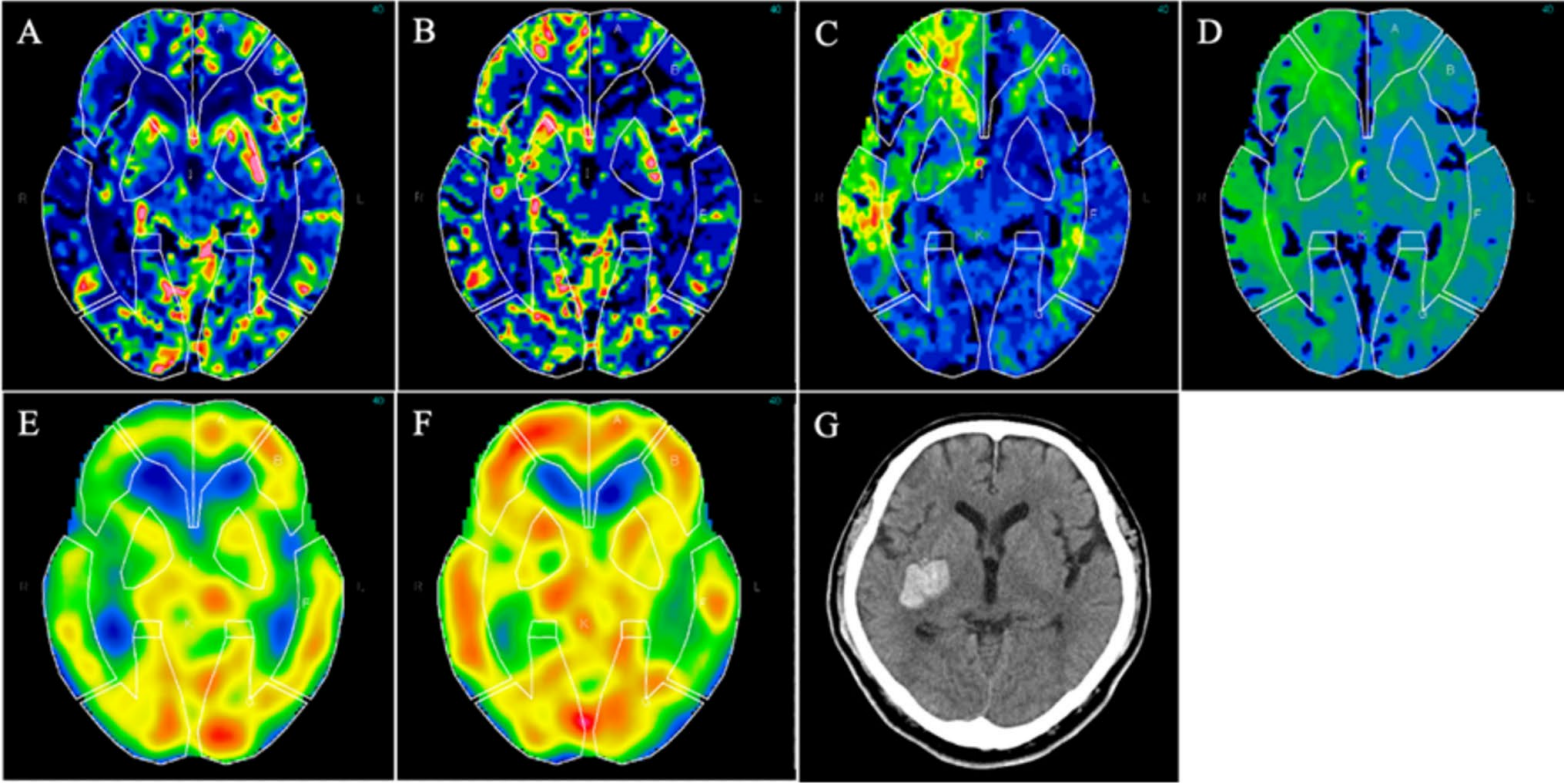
Imaging results of a 65-year-old man with a right carotid artery stenosis followed by intracerebral hemorrhage after CAS. Reconstructed maps of preoperative CTP showing a relatively decreased regional CBF (CBF ratio: 0.83) (A), increased regional CBV (CBV ratio: 1.24) (B), increased regional MTT (MTT ratio: 1.31) (C), and increased regional TTP (TTP ratio: 1.10) (D) in the right hemisphere. Preoperative ^123^I-IMP SPECT at rest showing decreased regional CBF (AI: 0.88) in the right hemisphere (E). Postoperative ^123^I-IMP SPECT at rest showing increased regional CBF (AI: 1.17) in the right hemisphere after CAS (F). The patient had cerebral hemorrhage in the ipsilateral basal ganglia on the postoperative day 3 after CAS (G)
